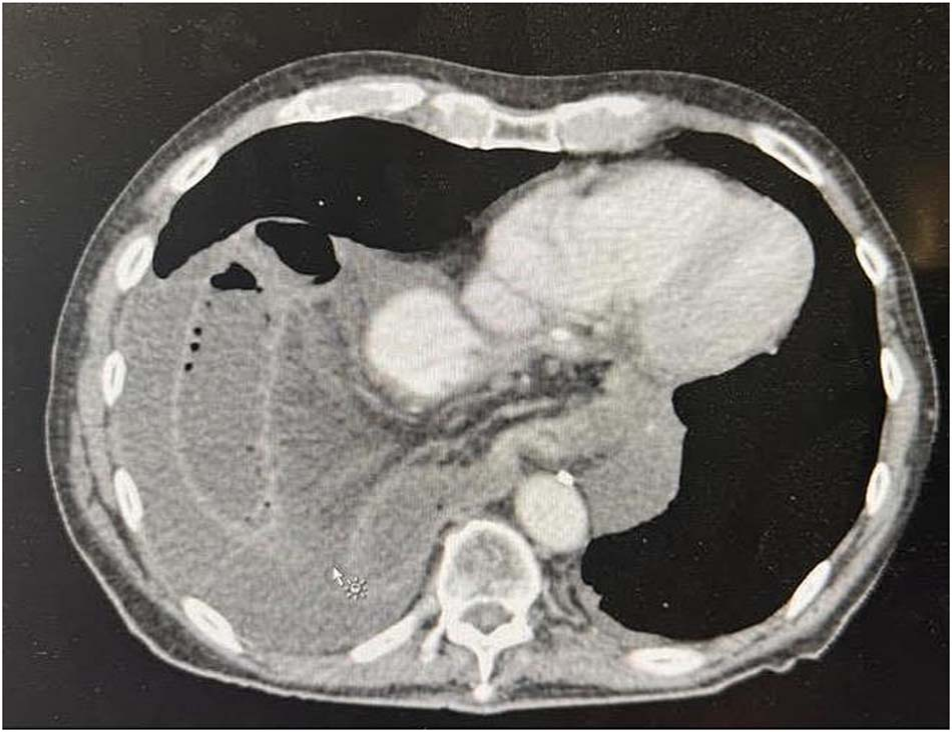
Preoperative CT image showing recurrent hiatal hernia with dilated small bowel in the right hemithorax, mesenteric edema, and surrounding fluid.
